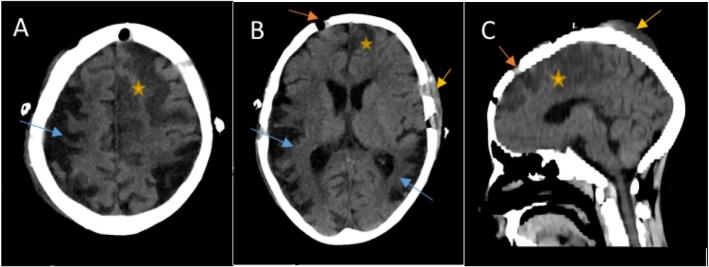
Selected axial brain CT scan images were done for our patient after surgery. They are showing new left frontoparietal (yellow stars in all images) cortical and subcortical low attenuating area denoting subacute ischemic injury in the territory of left ACA (Anterior cerebral artery). Blue arrows in A and B images refer to encephalomalacia areas secondary to old infarctions. Postoperative changes are noted as yellow arrows in B and C images are related to subgaleal hematoma and subcutaneous edema at site of surgery. While orange arrows at image same images show multiple skull bone defects.
